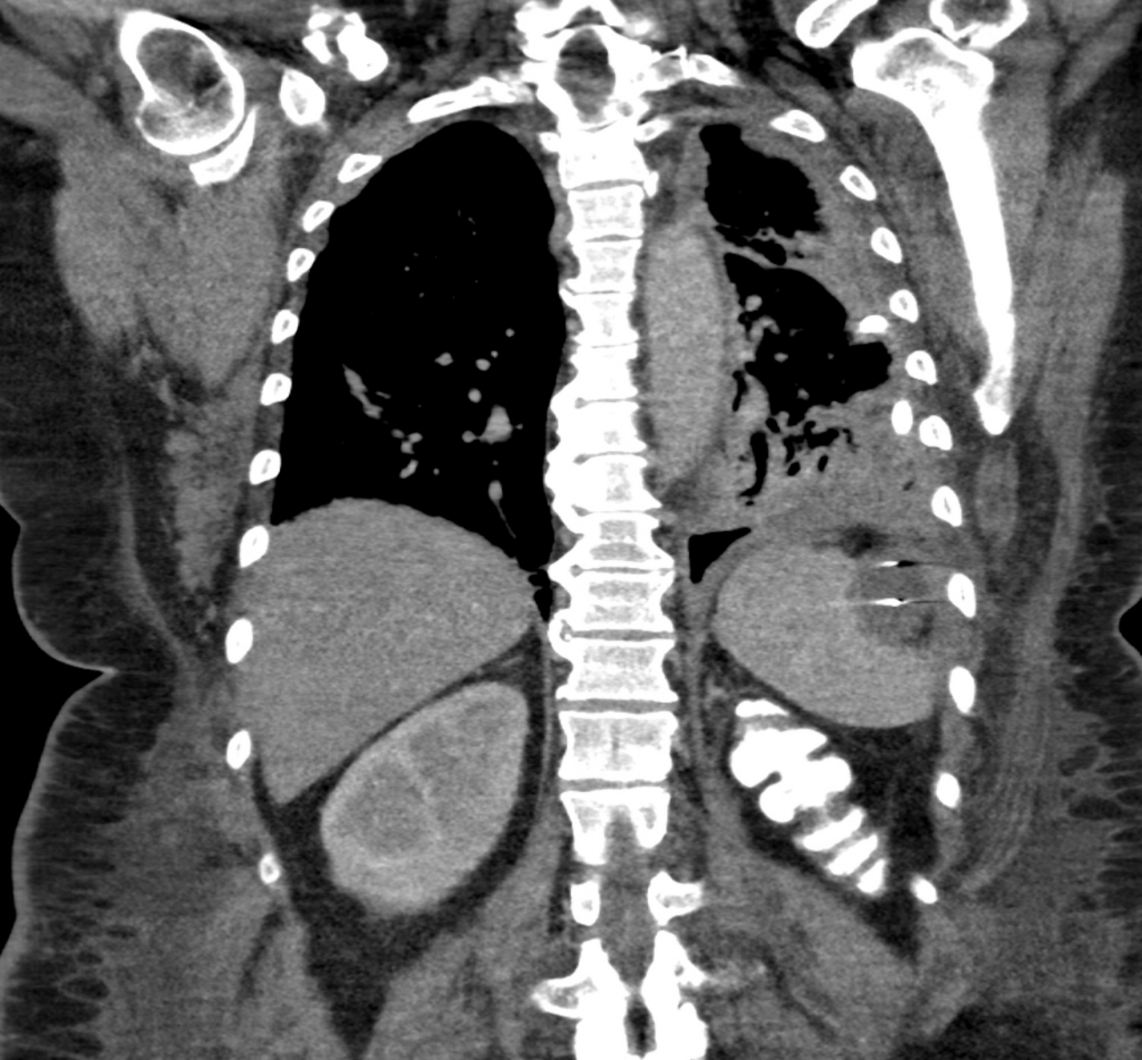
CT scan with oral contrast performed one day after esophagoduodenoscopy with endoscopic suturing of the fistula shows near-complete drainage of the left sub-diaphragmatic collection with residual gas There is no definite leakage of oral contrast into the left sub-diaphragmatic or pleural spaces. CT: computed tomography
